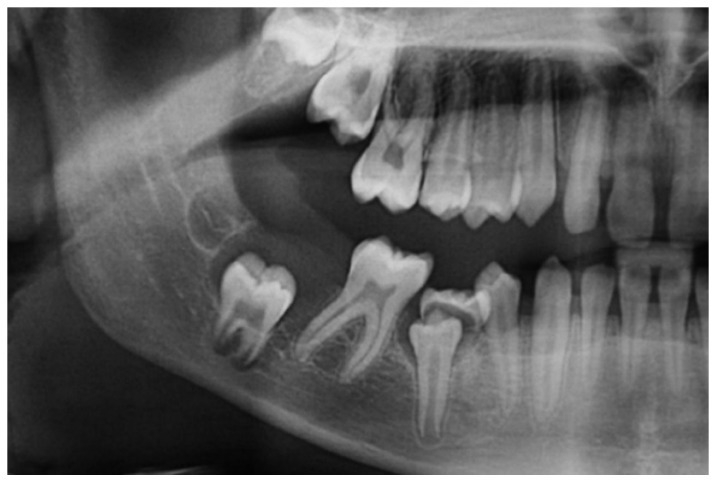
Panoramic radiography made at the age of thirteen: A unilocular well-defined radiolucent lesion (measuring around 4 mm) surrounding impacted mandibular right second molar.
